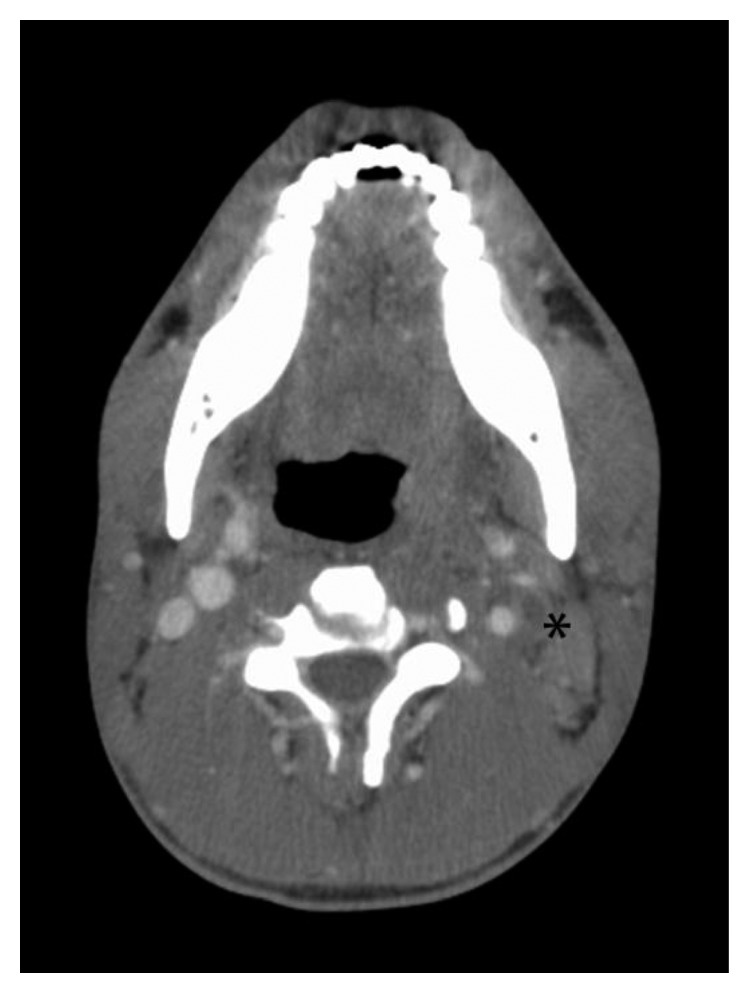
Contrast-enhanced CT scan of neck showing filling defect in left internal jugular vein (asterisk).
